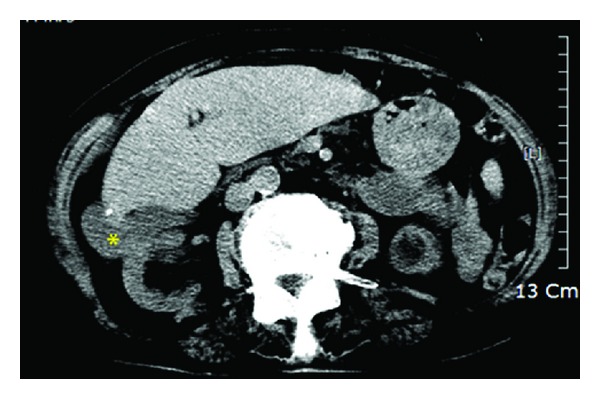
Axial plane of the abdominal CT examination revealed the ectopic position of the gallbladder (asterisk) which was laterally displaced.
